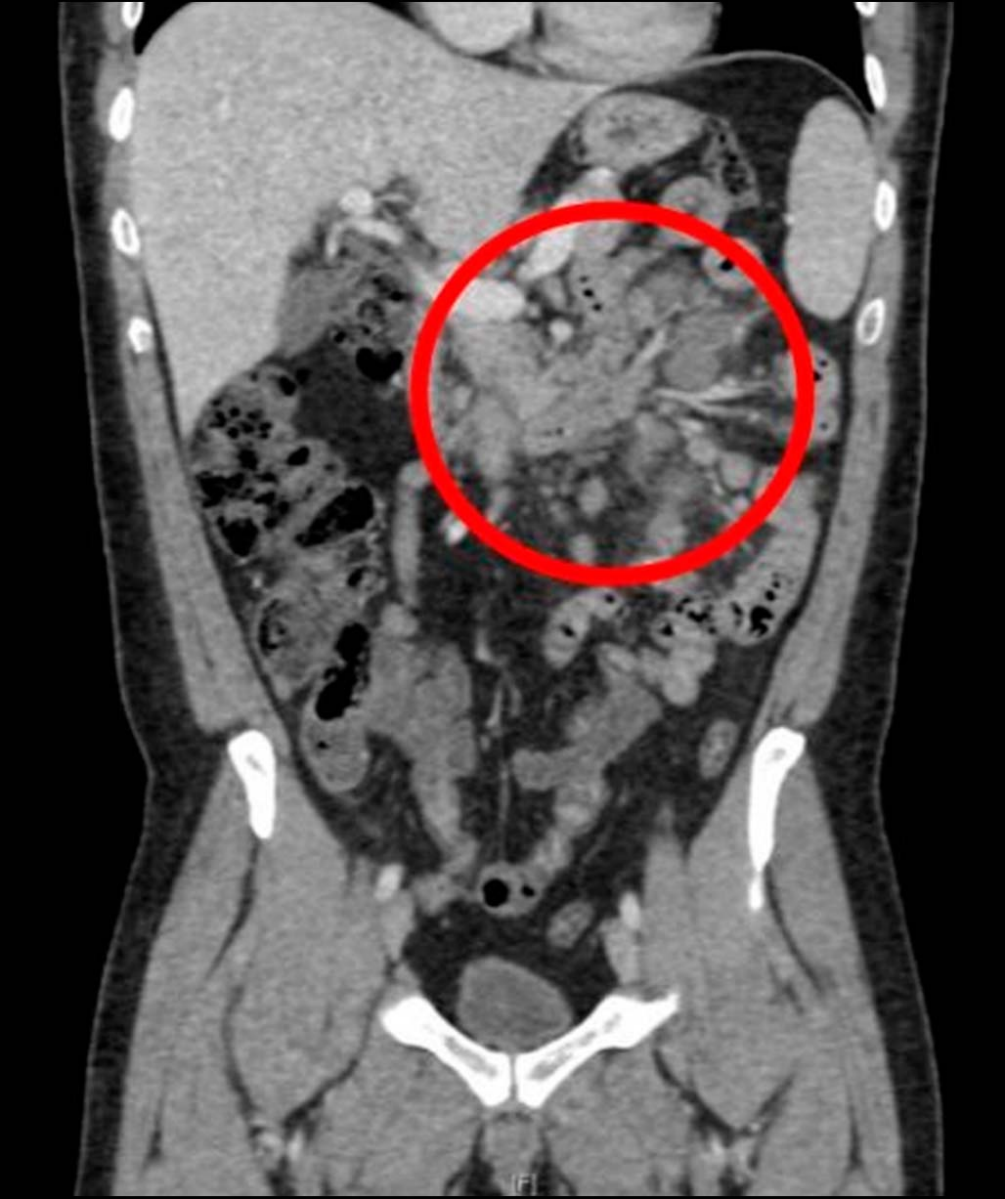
Abdominal lymphadenopathy noted on abdominal computed tomography, as indicated by red circle.
